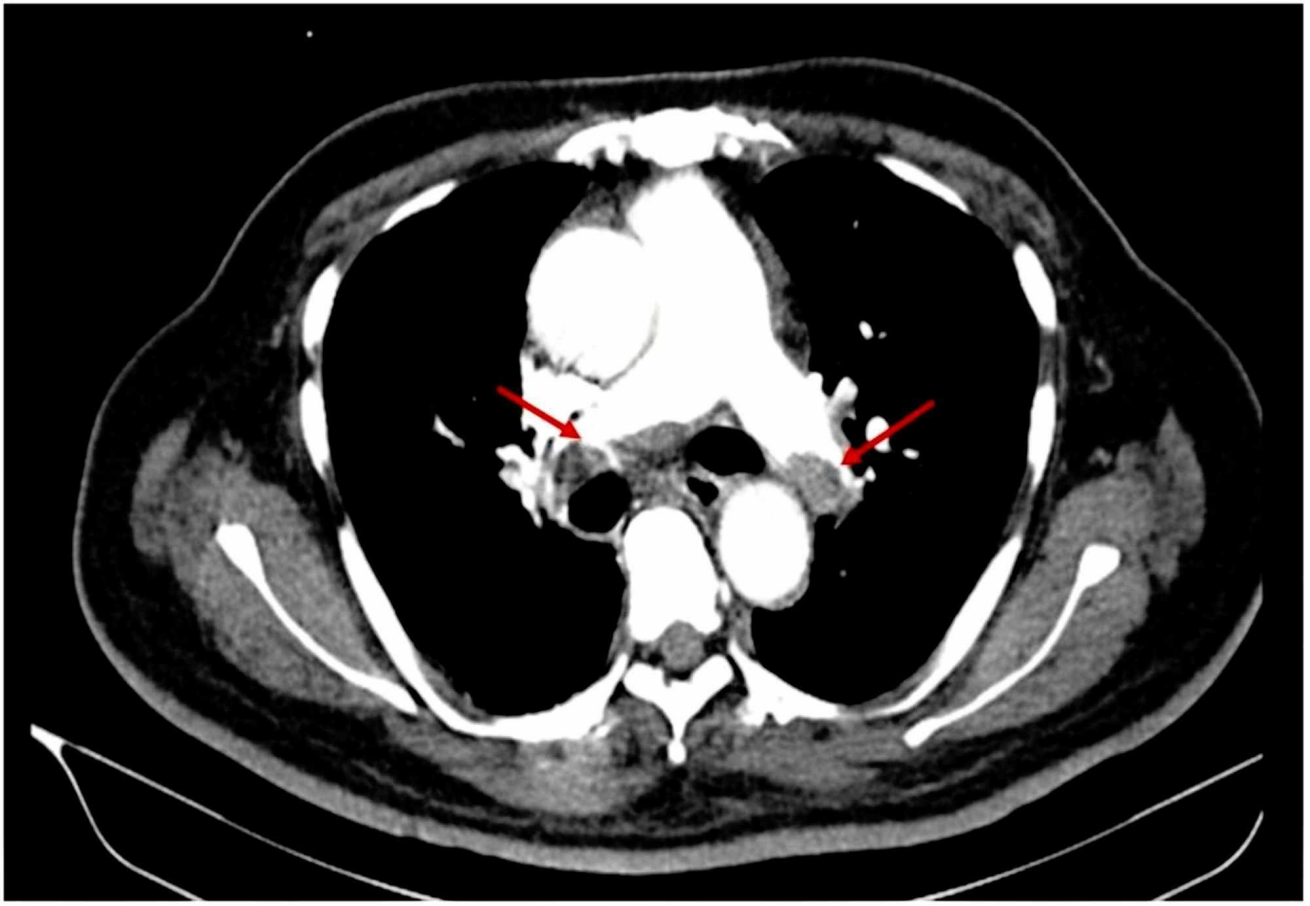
Bilateral Pulmonary Embolism (PE) CT angiography of the chest showing large filling defects in bilateral pulmonary arteries, segmental, and subsegmental arteries, suggesting massive PE.
